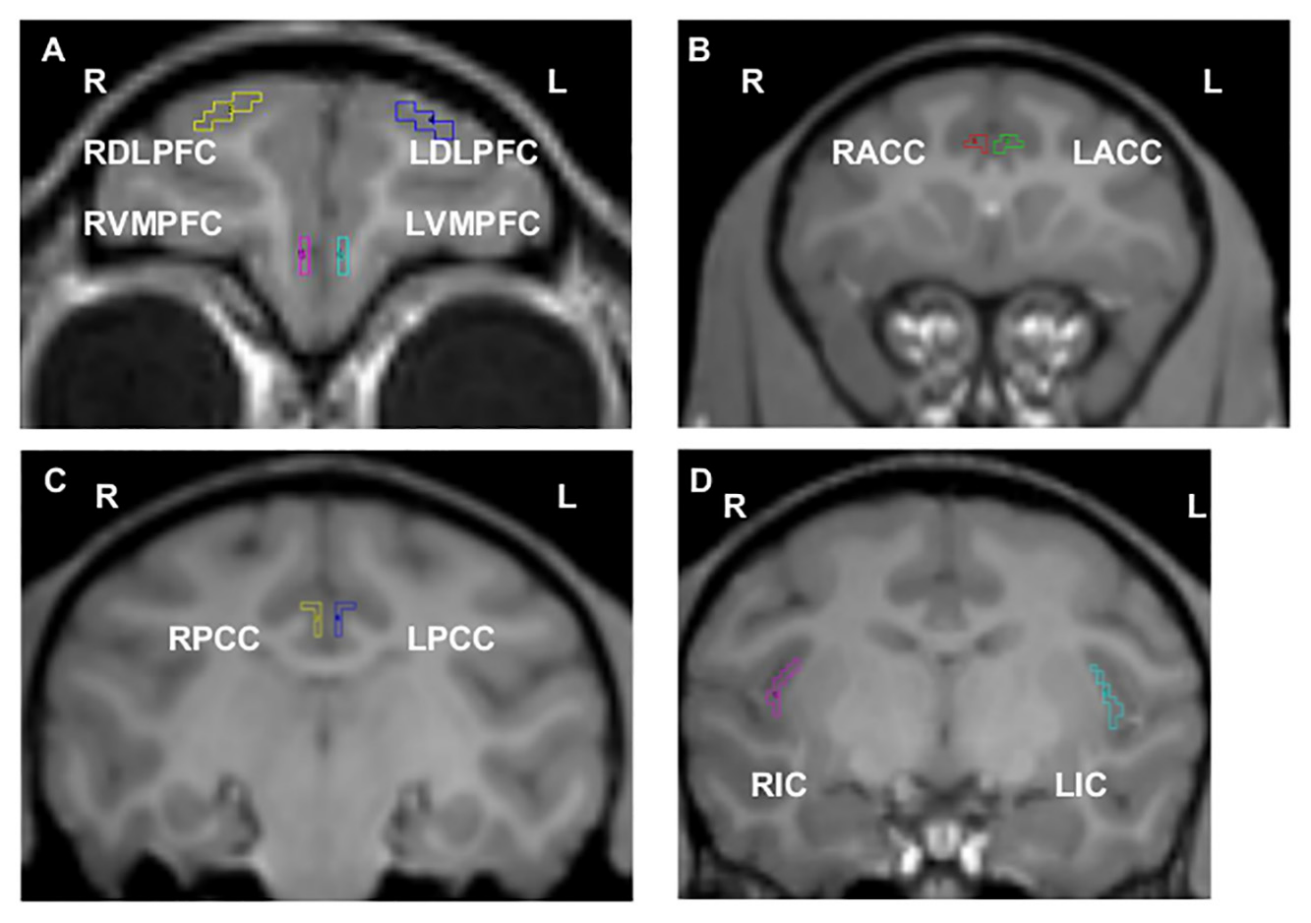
Regions of interest analyzed in brain are drawn on T1-weighted MR images. Region of interest (ROI) extended two additional coronal slices in the anterior and two in the posterior directions. ROIs are shown in right (R) and left (L) hemispheres: (A) dorsal lateral prefrontal cortex (RDLPFC, LDLPFC), and ventromedial prefrontal cortex (RVMPFC, LVMPFC), (B) anterior cingulate cortex (RACC, LACC), (C) posterior cingulate cortex (RPCC, LPCC) and (D) insular cortex (RIC, LIC).
